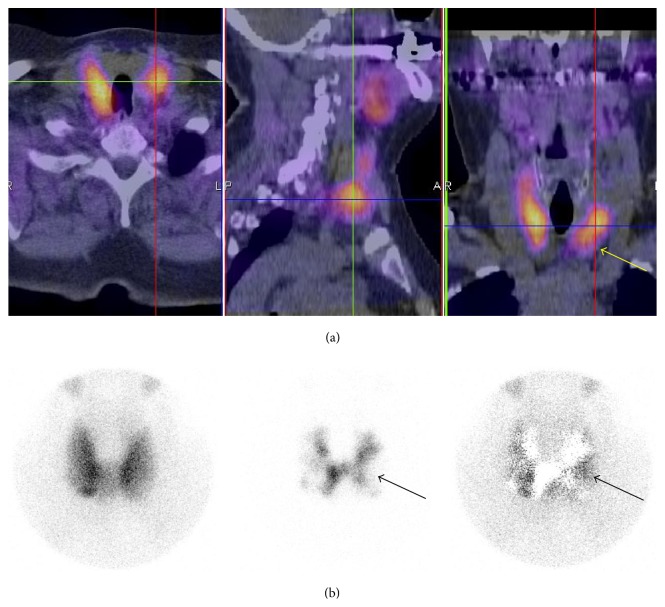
An example of a false-positive finding (case number seven in Table 3) on a ^99m^Tc-sestamibi/^123^I SPECT/CT due to an uneven iodine uptake and iodine-negative nodule on a left thyroid gland (arrows). (a) Fusion SPECT/CT images. (b) Anterior pinhole images (*left*: ^99m^Tc-sestamibi image;* middle*: ^123^I image;* right*: subtraction image).
